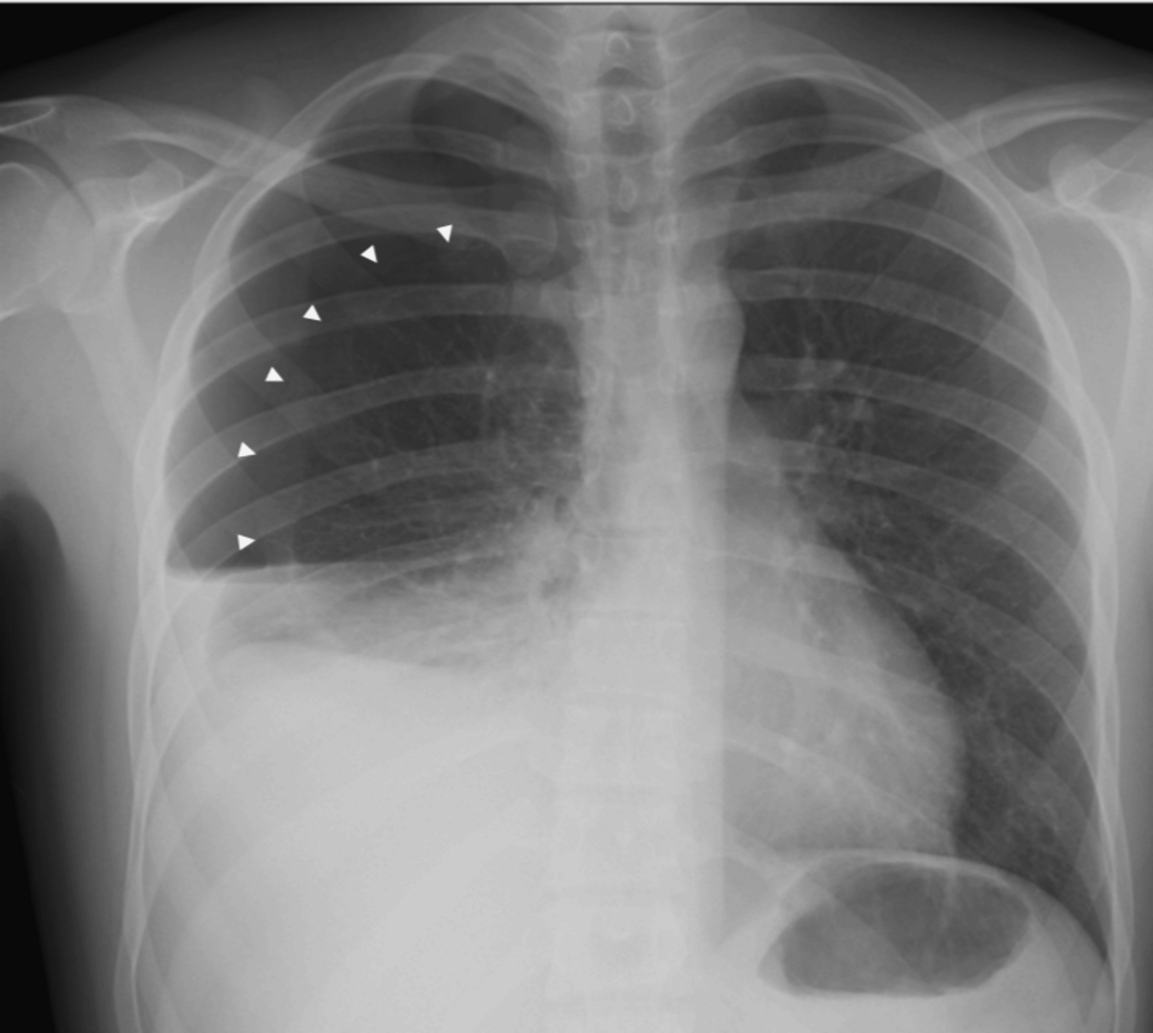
Chest radiograph at admission Posteroanterior chest radiograph demonstrating a right-sided pneumothorax with associated moderate pleural effusion. The lung is partially collapsed with a visible air-fluid level (white arrowheads outline the margin of the collapsed right lung).
